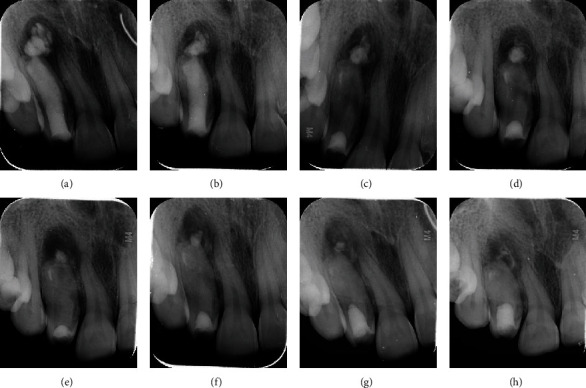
(a) Placement of injectable calcium hydroxide in the first visit. (b) Two-week follow-up. (c) Three-week follow-up. (d) Four-week follow-up. (e) Six-week follow-up. (f) Eight-week follow-up. (g) 10-week follow-up. (h) 12-week follow-up (upon every visit, calcium hydroxide dressing was replaced with freshly mixed calcium hydroxide powder in saline. Extruded calcium hydroxide resorption and periapical healing can be noted in each visit).
